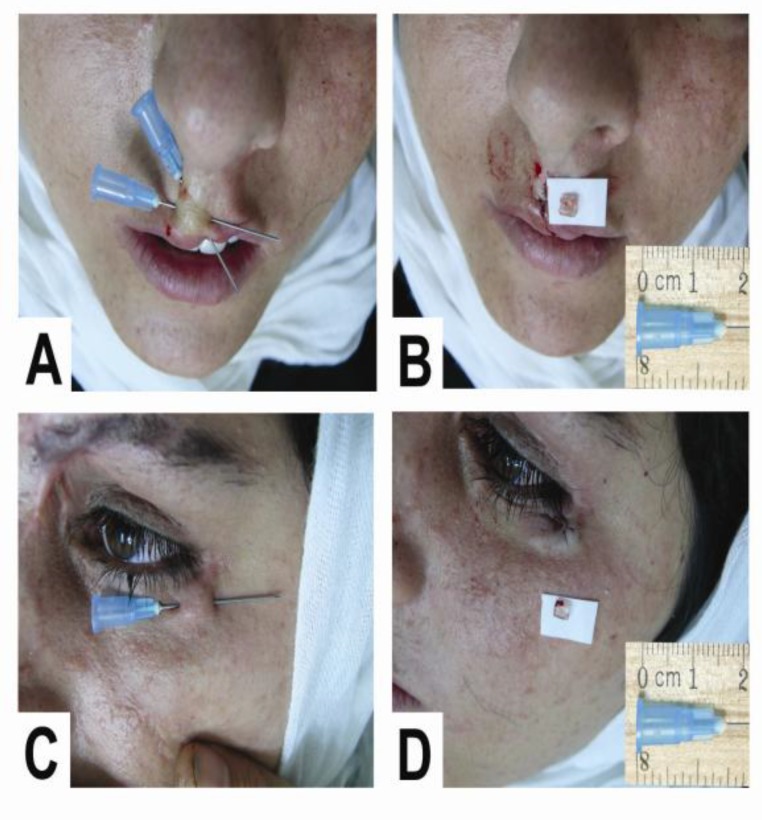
Removal of an STFB in a 27-year old woman with upper lip and lower lid foreign body sensation 14 months after a car accident, with multiple traumas and several facial scars. Radiographs obtained were negative
